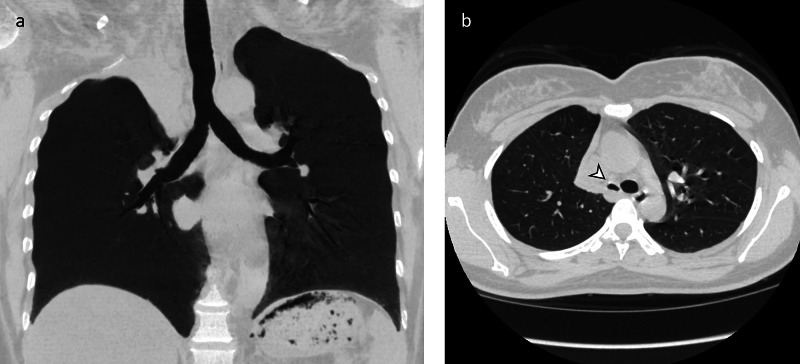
Absence of right upper lobe bronchus. Minimum-intensity projection CT chest (a) demonstrates the absence of the right upper lobe bronchus. Axial CT chest (b) demonstrates narrowing of the right main bronchus relative to the left main bronchus (arrowhead).
